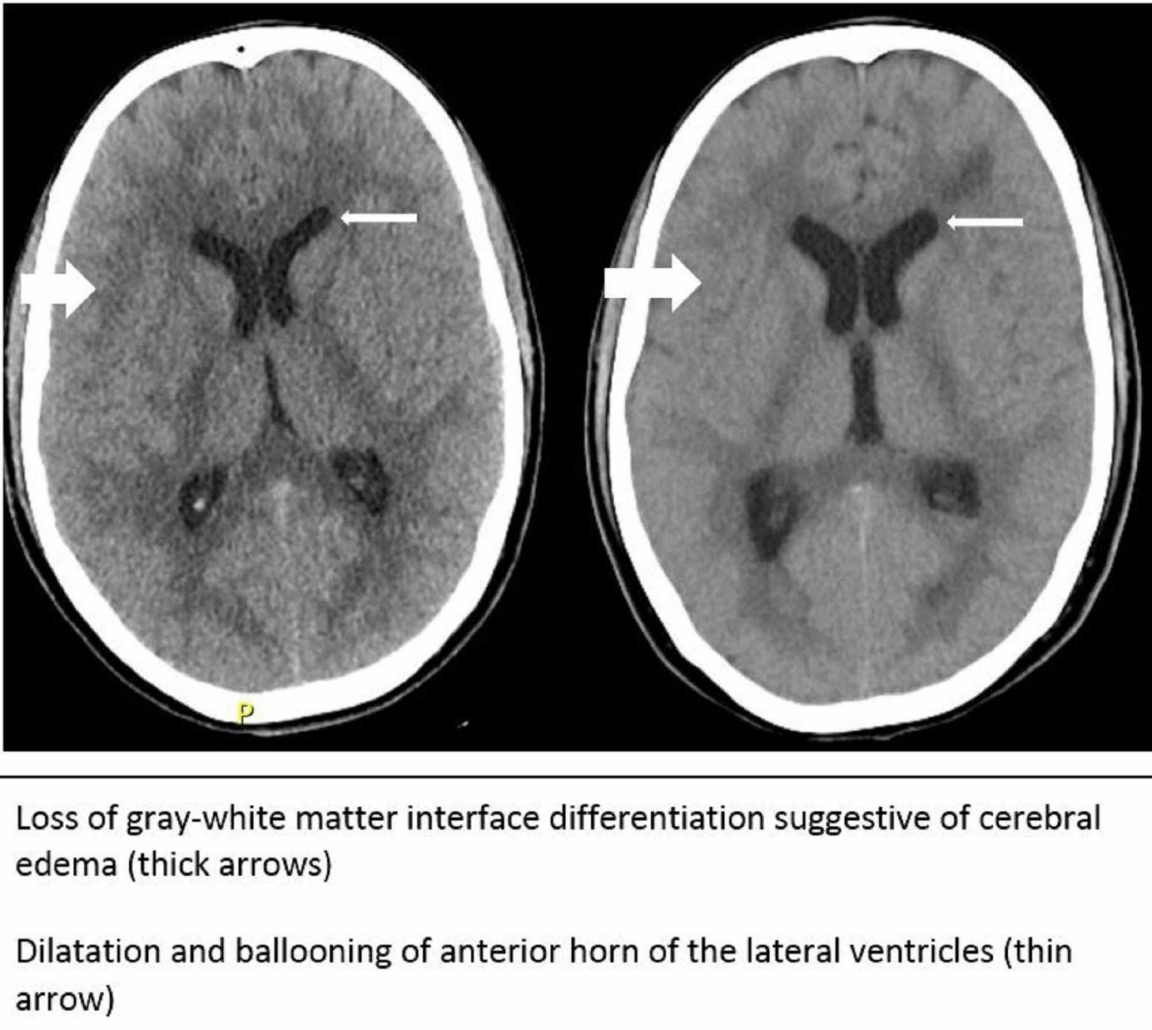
Development of cerebral edema and hydrocephalous; (left CT scan) before and (right CT scan) evaluated by neurosurgeon and radiologist.
